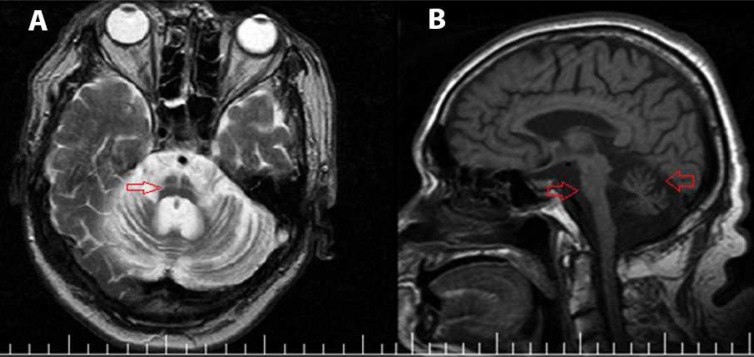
A: Axial T2-weighted imaging shows severe cerebellar atrophy and a typical hot cross bun sign in pons (red arrow). B: Sagittal T1-weighted magnetic resonance imaging shows severe cerebellar and pontine atrophy (red arrows).
